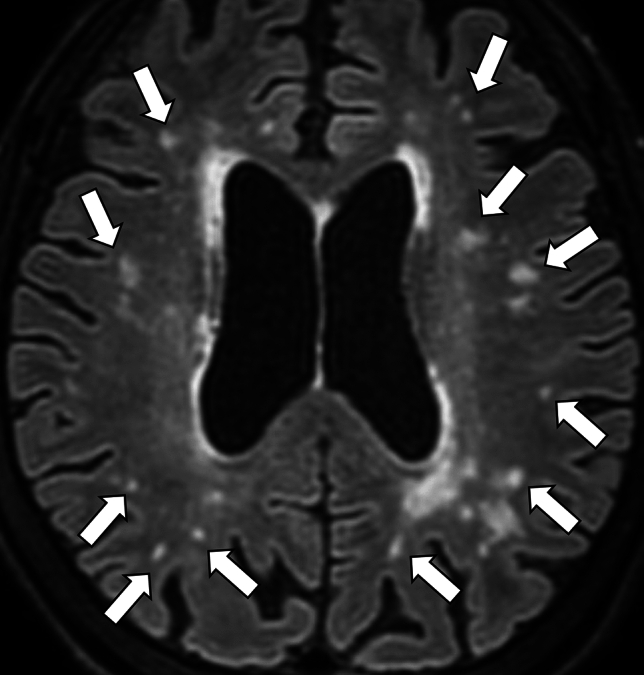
An 89-year-old male with probable CAA. MMSE score is 24. 3D FLAIR shows subcortical hyperintense areas in a multispot pattern (arrows)
